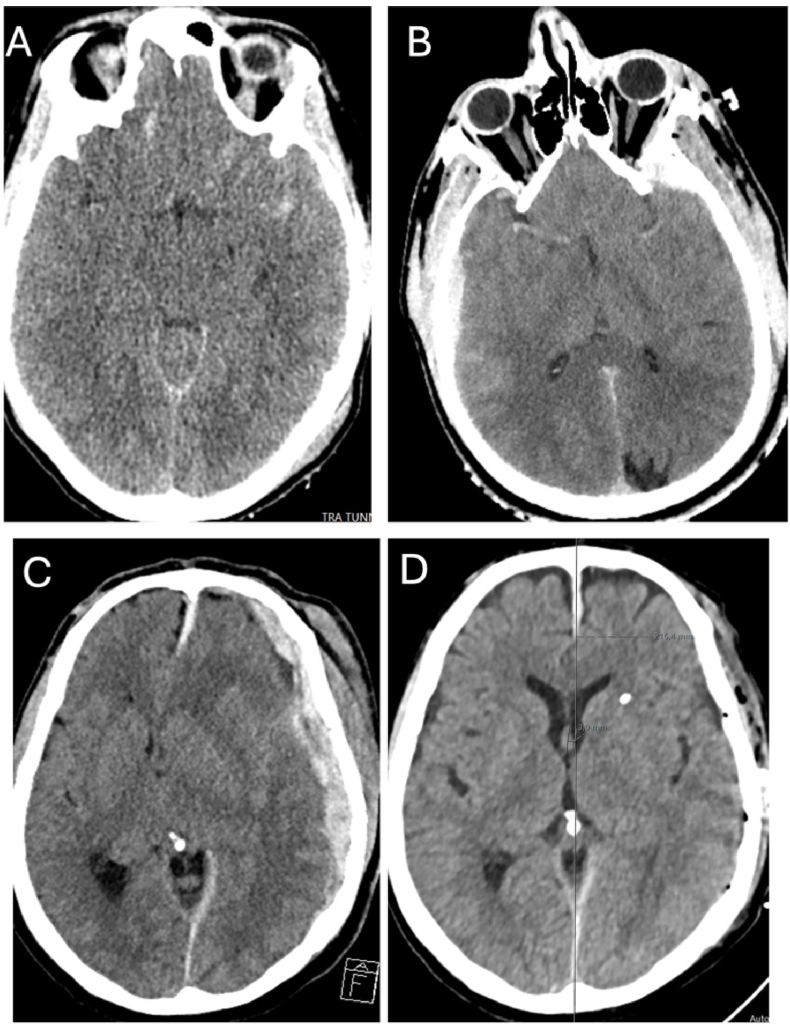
CT scans of us-TBI patients achieving a good outcome (Glasgow outcome scale extended, GOSE, 7-8). In A), a 19-years old female was found 10m from her car after a motor vehicle accident at an approximate speed of 90 km/h. On assessment, she was GCS 5 with bilaterally small unresponsive pupils. On CT she had signs of widespread traumatic axonal injury. She had an uneventful neurocritical care course, received a tracheostomy and achieved an excellent recovery after a lengthy neurorehabilitation. B) A 26-years old male involved in a motorcycle accident and was GCS 5at the scene of the accident. He had on CT bilateral epidural hematomas and a complex fracture system involving the bilateral skull base, orbital fractures, bilateral temporal bone fractures and a zygomatic arch fracture on the left side. He received ICP monitoring and wound revision and could be extubated on day 3. C-D) A 60-year-old male was beaten and found outside at a GCS of 9-10. At the ER of the local hospital, 20 min away from our neurosurgical department, he rapidly deteriorated to GCS 3 and dilated left pupil. He was taken to our department for emergent surgery and evacuation of the left-sided acute subdural haematoma. Already at one day post-surgery (D) he was GCS 14-15, with normal pupils and could be extubated.
